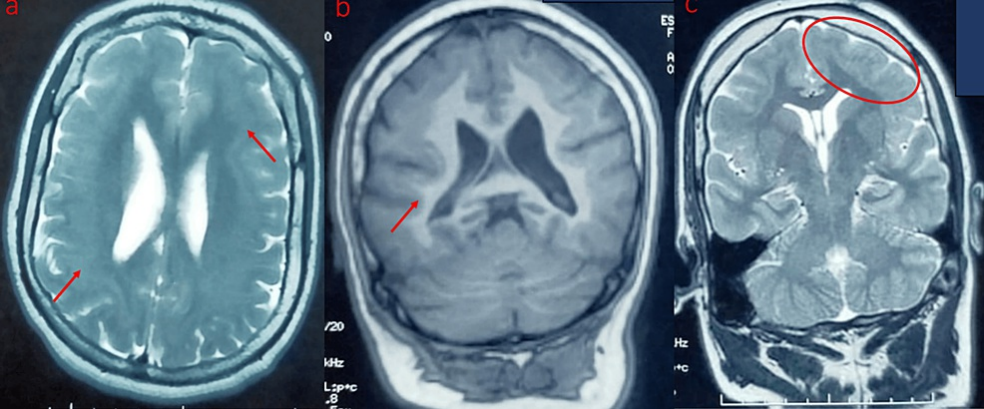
MRI brain axial T2 (a), coronal T1 (b), and coronal T2 (c) sequences showing characteristic subcortical heterotopic bands of gray matter (straight red arrows) with location and signal intensity parallel to the pachygyric overlying cortex (red circle).
